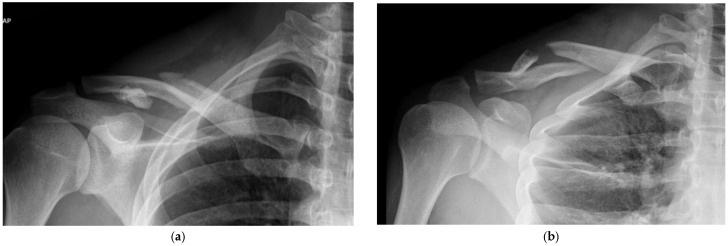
A 22-year-old male patient was diagnosed with a right clavicle mid-shaft fracture during sports activity. Fracture findings corresponding to AO/OTA classification 15.2 C with multiple fragments were confirmed on clavicle AP (a) and lordotic view X-rays (b).
